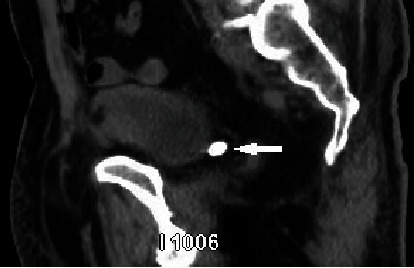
CT image on sagittal view in 2023 shows a pelvic stone posterior to the bladder (white arrow).
